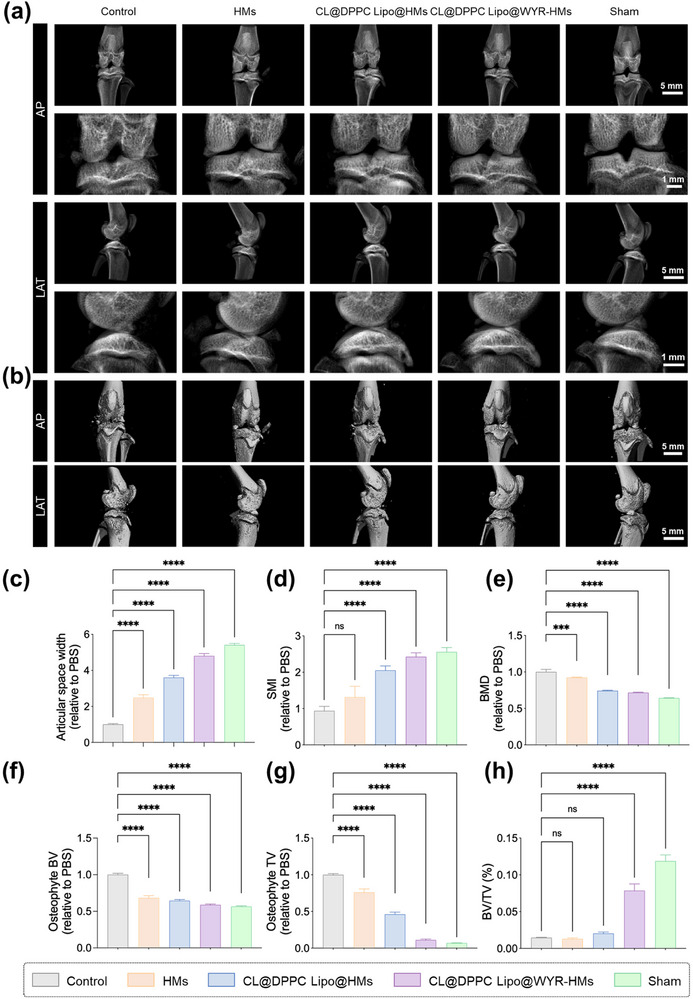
Micro‐CT evaluation. a) The measured X‐ray images of the obtained knee joint. b) The micro‐CT images of the obtained knee joint. c) The relative JSW. d) The measured relative structure model index (SMI). e) The relative osteophyte volume (BMD). f) The relative tissue volume (TV). g) The relative bone volume (BV). h) The relative BV/TV. (*p* < 0.0001).
